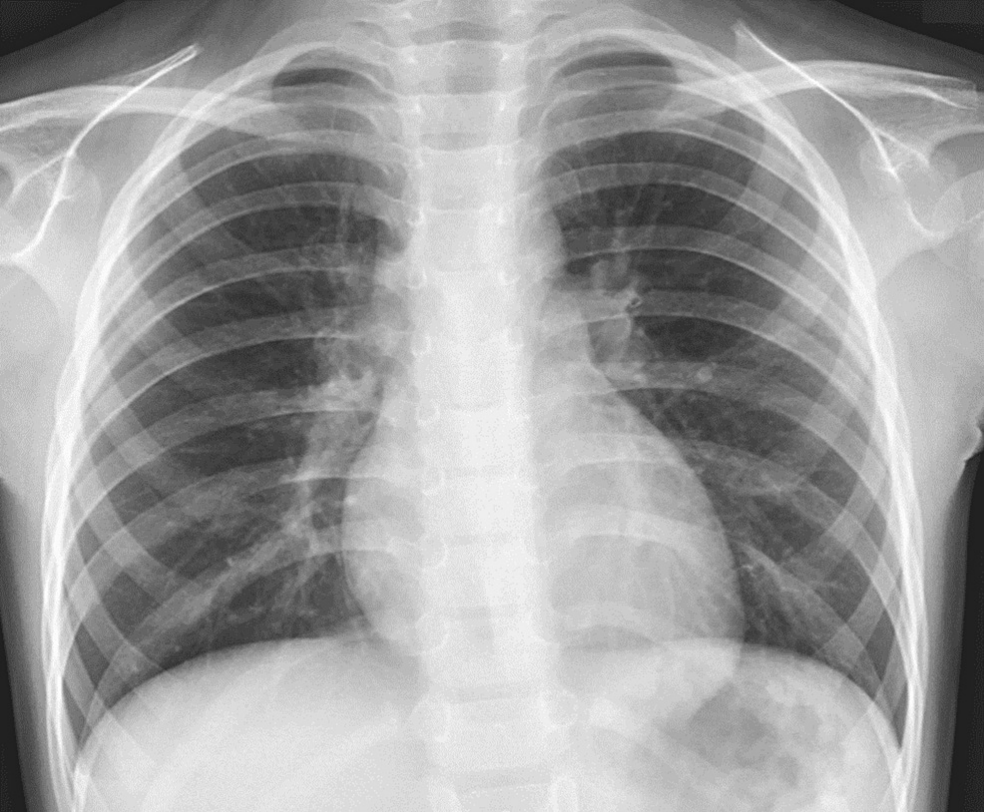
Chest X-ray showing no signs of respiratory tract infection.
